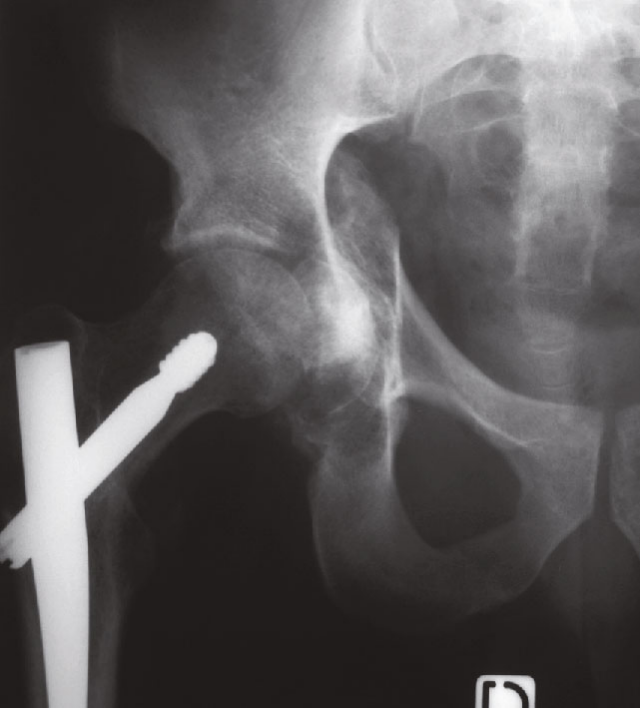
Postoperative X-ray of the intra-articular buttress perfectly correcting the femoral head acetabular protrusion.
